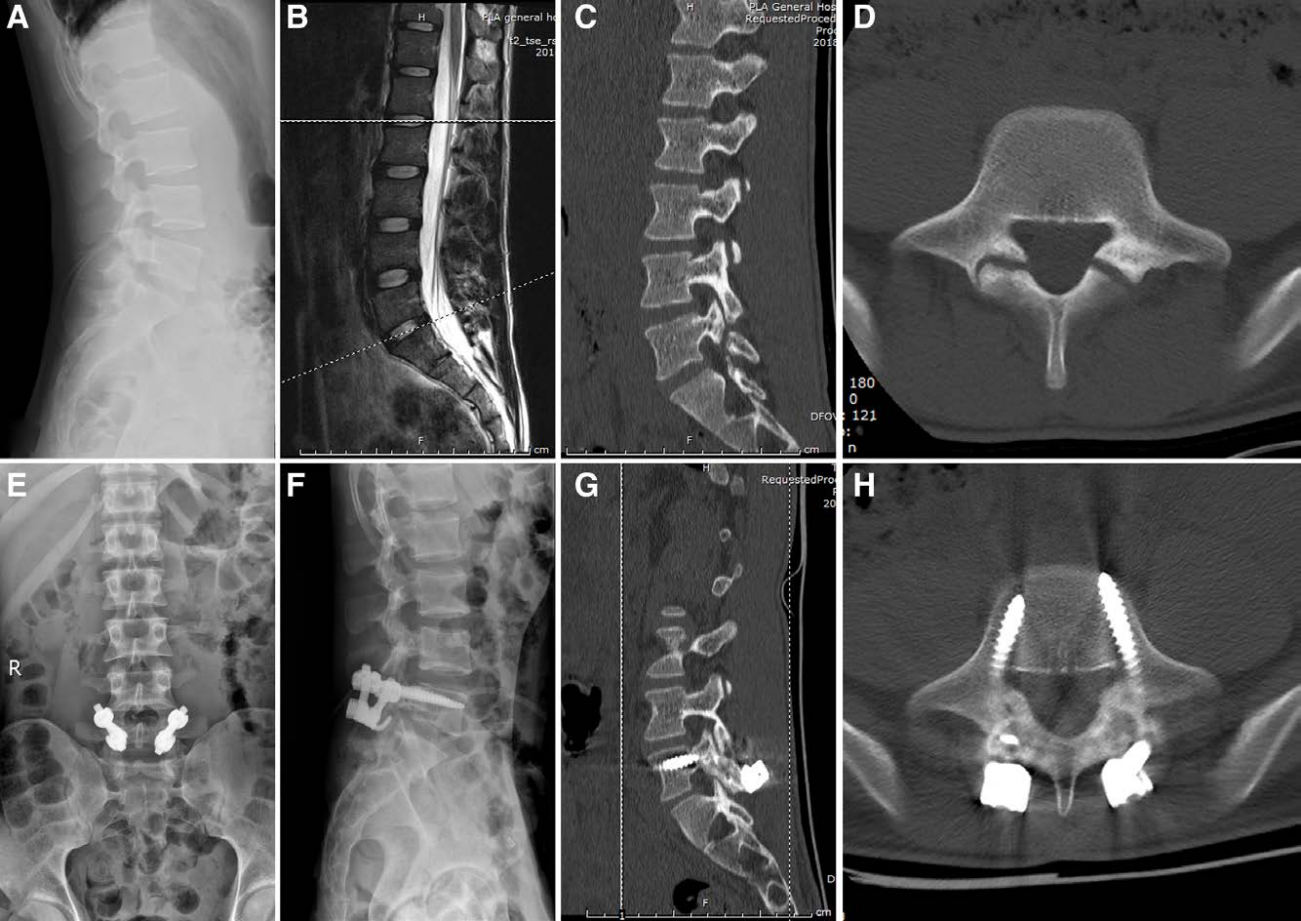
A 19-year-old man with a symptom of lower back pain for 1 year. (A) Preoperative lateral X-ray of the lumbar spine shows L5 spondylolysis. (B) Preoperative sagittal MRI of the lumbar spine shows no significant disc degeneration. (C and D) Preoperative sagittal and axial CT shows bilateral pars defect in the L5 isthmus. (E and F) Three days after the operation, the X-ray film shows a good internal fixation position. (G and H) Sagittal and axial CT shows bilateral isthmus bony healing at 1 year after surgery. CT = computer tomography, MRI = magnetic resonance imaging.
